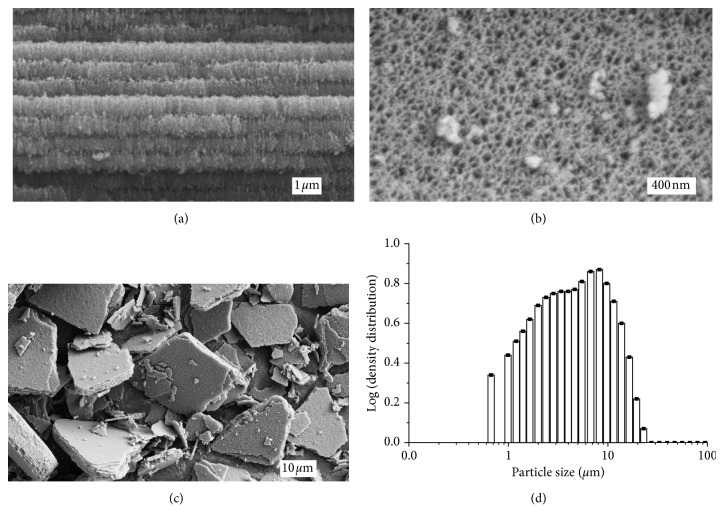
(a)–(c) FESEM images produced of the mesoporous THCPSi structure of the inactive reference substrates. (a) A cross-sectional view of the layer-like structure of the mesoporous surface. Each layer has an approximate thickness of 200 nm. (b) Image taken from above the structure depicts the inlets of the pores from the top. (c) Image produced of the mesoporous THCPSi particle distribution after ultrasonication. (d) Size distribution of the THCPSi reference particles obtained by laser diffraction.
